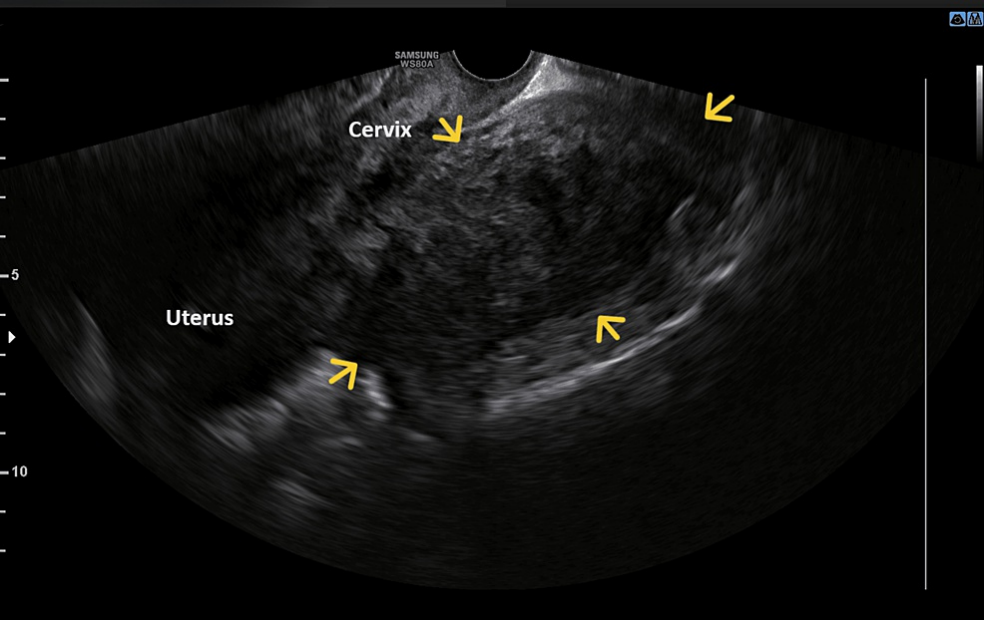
Transvaginal ultrasound of the posterior pedunculated extracervical leiomyoma of the uterus The demarcation (yellow arrows) of a large echogenic mass with solid elements, which occupies the pouch of Douglas, is indicated
